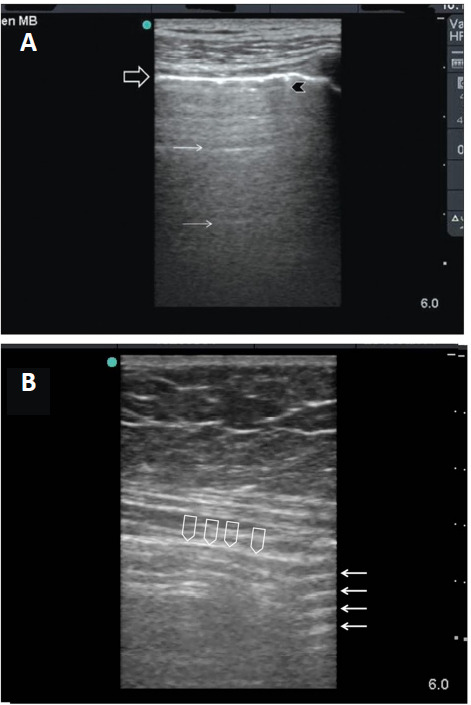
Reverberation artifact of the lung and free intraperitoneal air. (A) Reverberation artifact of the lung occurs as ultrasound waves bounce between the transducer and the pleura. The pleura is shown as a hyperdense white line (black arrow). The reverberation lines (white arrows) represent the repetition of the pleural line. A comet tail artifact is shown (black arrowhead). Reprinted with permission from Abu-Zidan et al.^5^ (B) Sonographic findings of free intraperitoneal air. An enhanced peritoneal stripe sign (arrowheads) does not move with respiration. The reverberation artifact consists of hyperechogenic parallel lines with equal distance between them (arrows). Reprinted with permission from Khan et al.^8^
